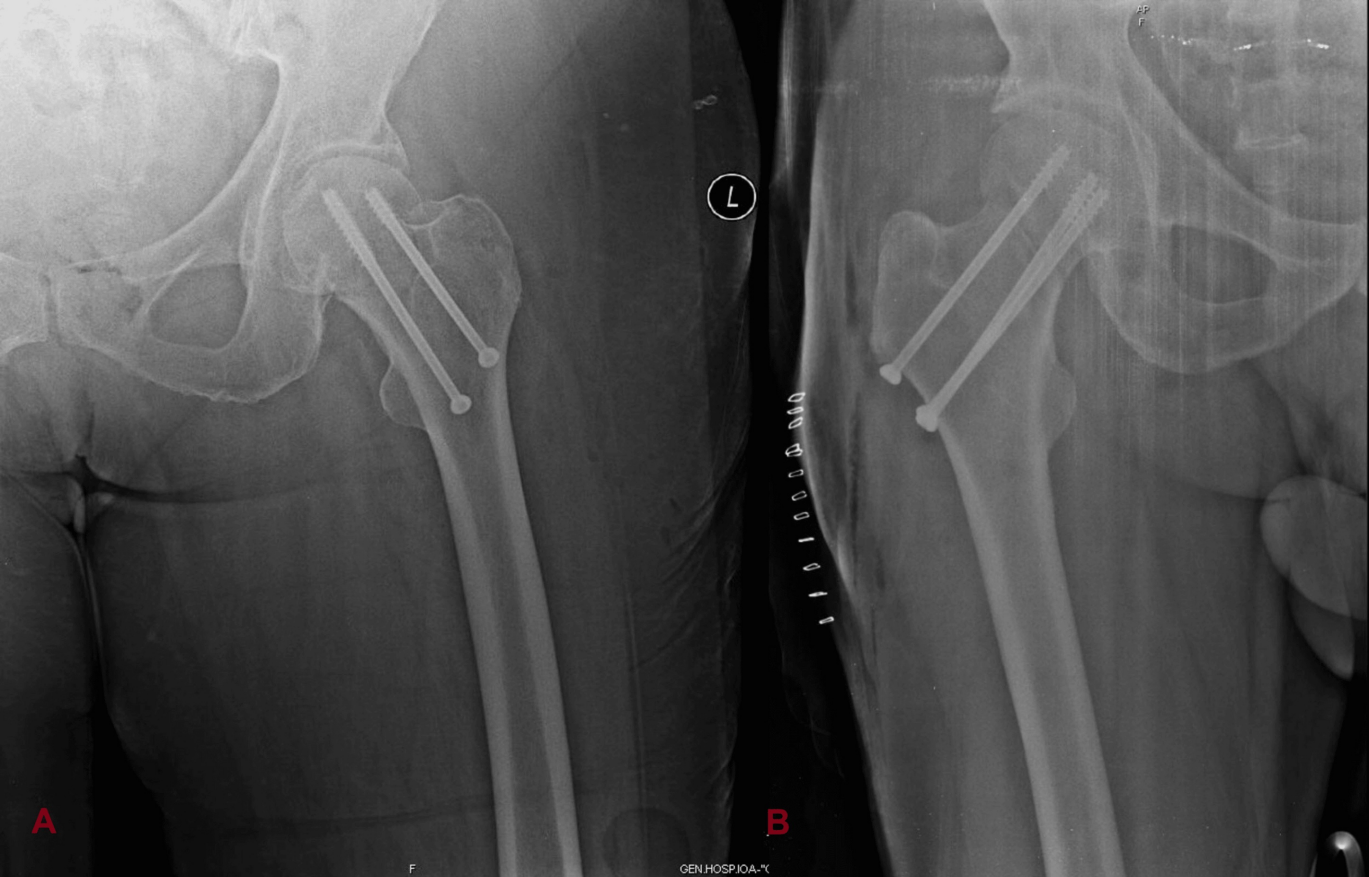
X-rays demonstrating the fixation of FNFs with cannulated screws A: Use of two screws. B: Use of three screws. FNF, femoral neck fracture
